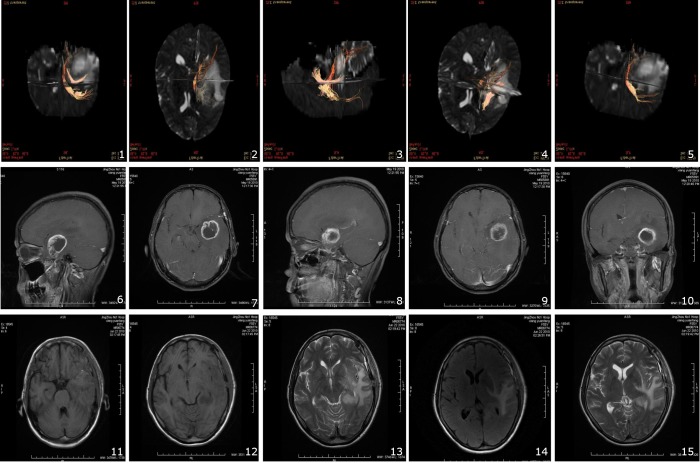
MR DTI (Figure 1-5) can assess the completeness and consistency of the white matter fibers, “depression, edema, infliction, and damage” by the tumor. The pyramidal tracts and the deep white matter were partially invaded and damaged. MRI (Figure 6-15), preoperative axial (Figure 7, 9), sagittal (Figure 6, 8), and coronal (Figure 10) T1-weighted enhancecd image showed tumor were hyper-signal with sharp border. Marked edemas surrounded the location at the medial temporal base and the insular lobe. Postoperative axial T1-weighted image (Figure 11, 12), T2-weighted image (Figure 13, 15) and T2 flair image (Figure 14) showed total tumor removal with mild edema surrounding.
